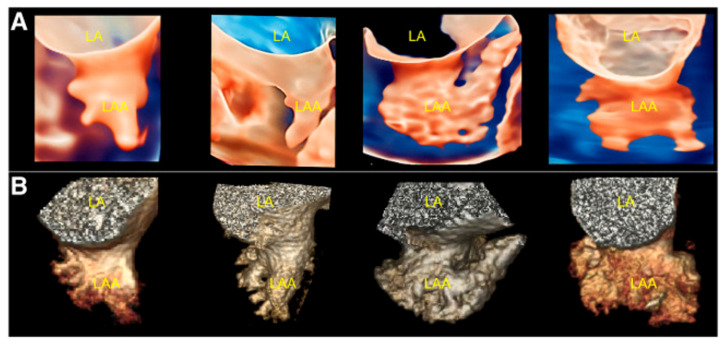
Comparison of TrueVue Glass and CT for displaying different morphologies of LAA. (A) From left to right, TrueVue Glass shows cactus, windsock, chicken wing, and cauliflower types of LAA. (B) From left to right, CT display of the above morphological types of LAA are shown. CT: Cardiac computed tomography; LA: Left atrium; LAA: Left atrial appendage. Adapted with permission from Sun et al. [27].
